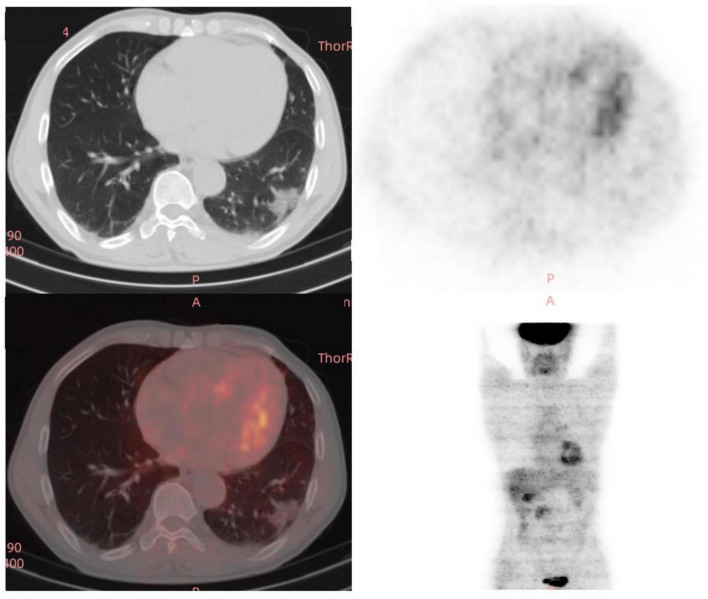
A total of 18 fluoro-D-glucose positron emission tomography/computed tomography (18F-FDG-PET/CT) images of the chest in the third year (12/29/2023) of re-examination of the patient.
